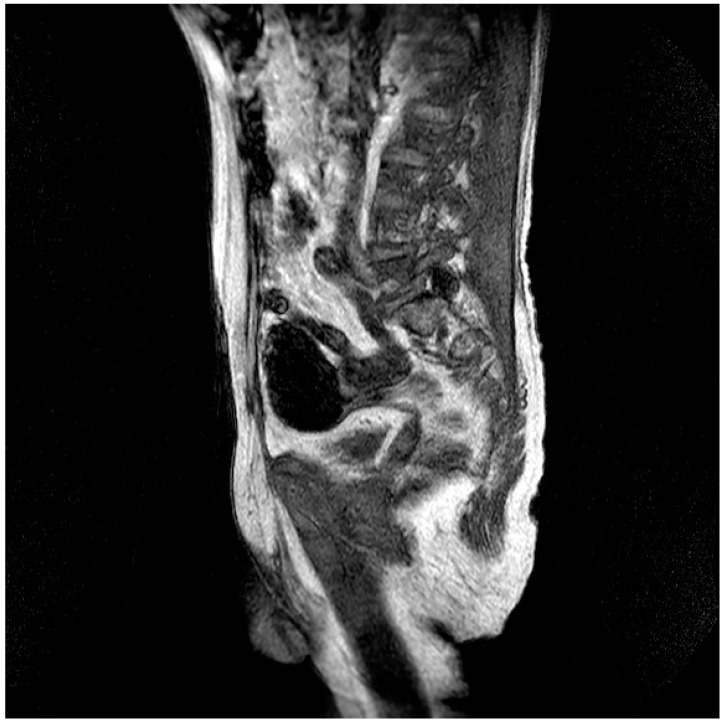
Evidence of an extensive epidural spinal cord hematoma on spinal MRI (Table 1, patient 7).
